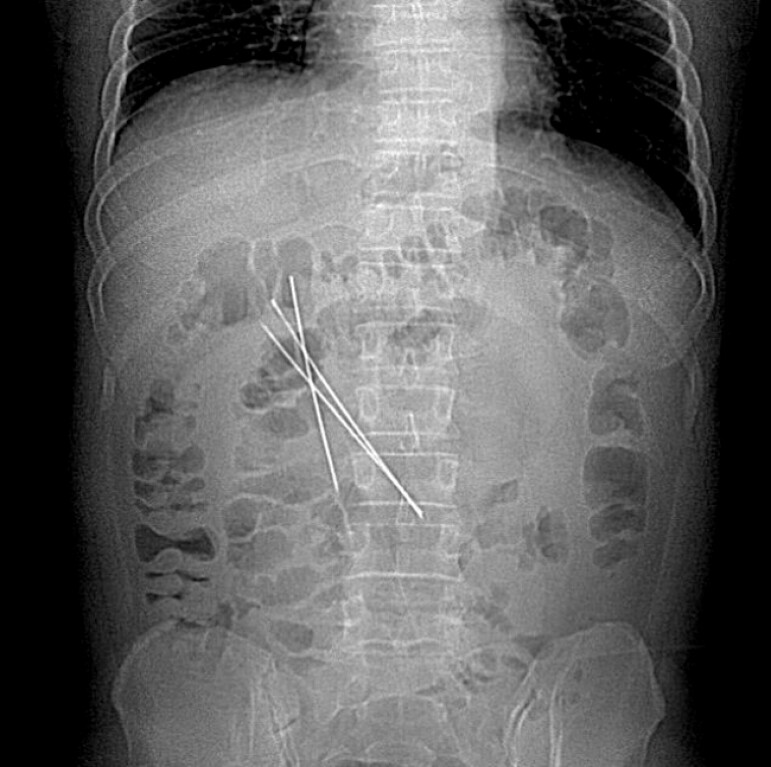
X-ray revealed three metallic objects in the descending and horizontal portions of the duodenum.
